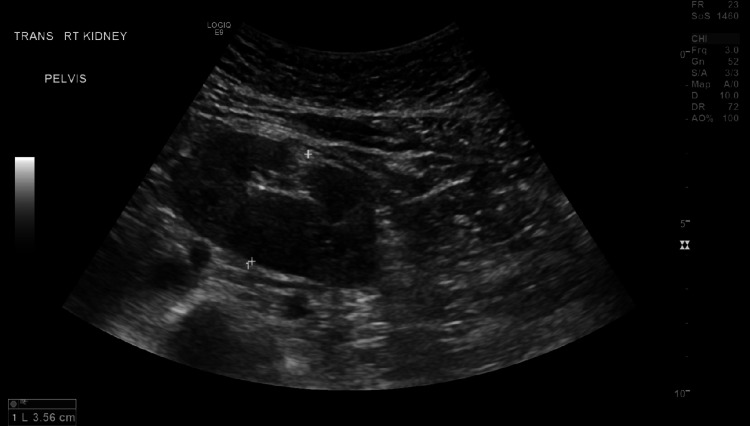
Ultrasound showing right moiety measuring 9.6 cm. There is no hydronephrosis or shadowing renal calculus. Parenchymal echogenicity is within normal limits.
